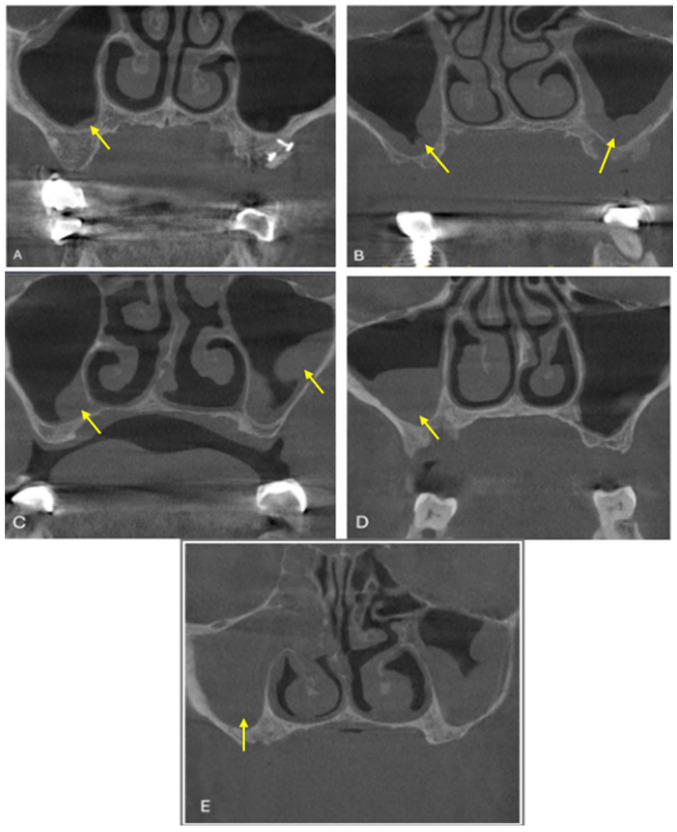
Maxillary sinus pathologies on coronal CBCT images (a yellow arrow indicates each pathology). (A) Localized mucosal thickening in the right maxillary sinus and a healthy left maxillary sinus; (B) generalized mucosal thickening in both the right and left maxillary sinuses; (C) polypoid mucosal thickening in the right and left maxillary sinuses; (D) partial opacification of the right maxillary sinus; and (E) total opacification of the right maxillary sinus.
